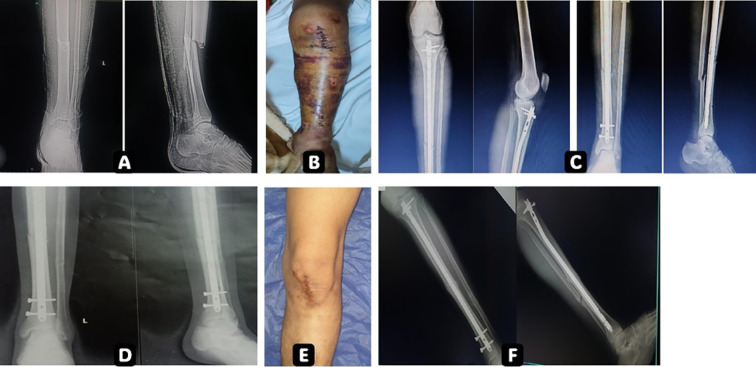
Representative case managed with IMN via MLTP. A) Preoperative anteroposterior and lateral radiographs of a left tibial shaft fracture. B) Clinical photograph showing the first postoperative dressing. C) Immediate postoperative anteroposterior and lateral radiographs. D) Three-month follow-up anteroposterior and lateral radiographs. E) Clinical photograph at 6 months showing the surgical wound. F) Six-month follow-up anteroposterior and lateral radiographs.
